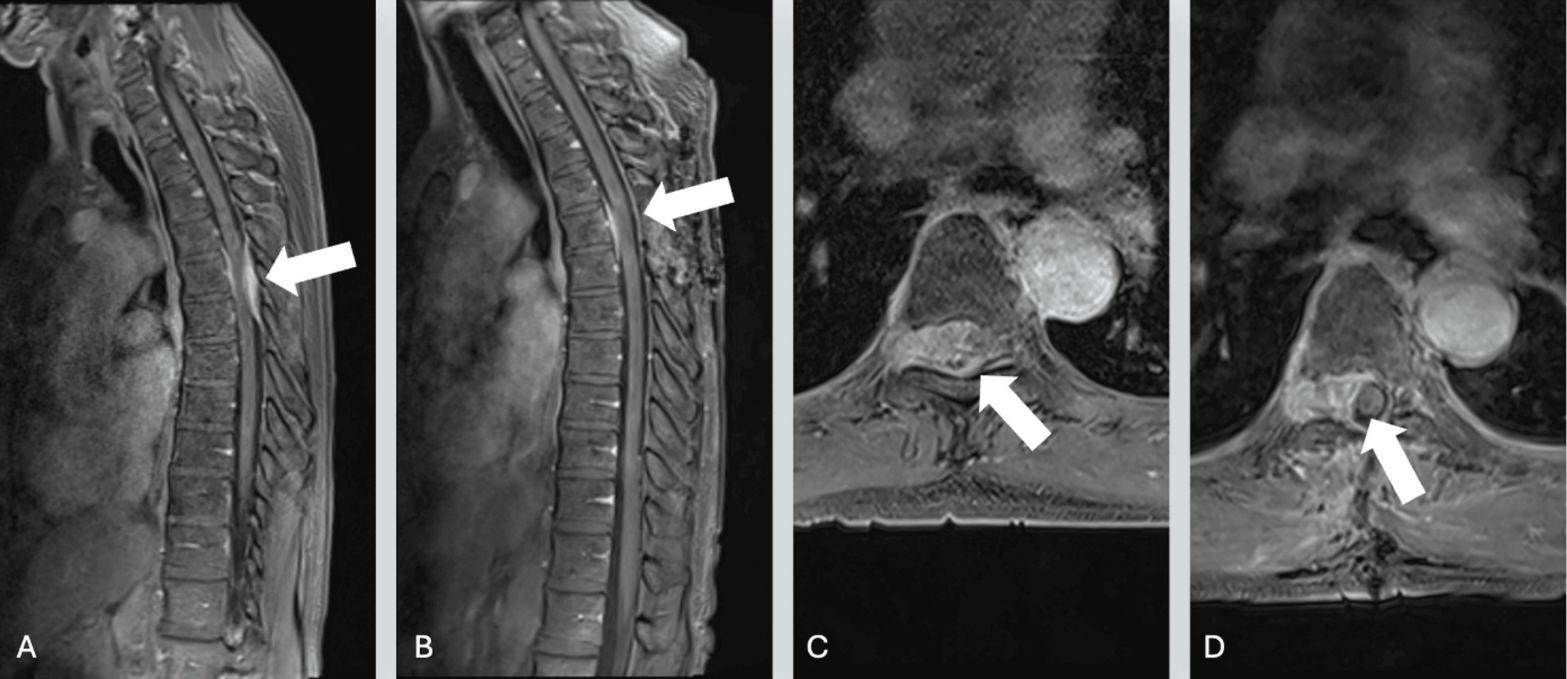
Sagittal magnetic resonance imaging (MRI) of the thoracic spine displaying the lesion at T5-T6 (A) and subsequent postoperative sagittal imaging acquired one day after resection (B). Axial MRI revealing a lesion at the T5-T6 region preoperatively (C) with a subsequent axial MRI one day postoperative (D).
